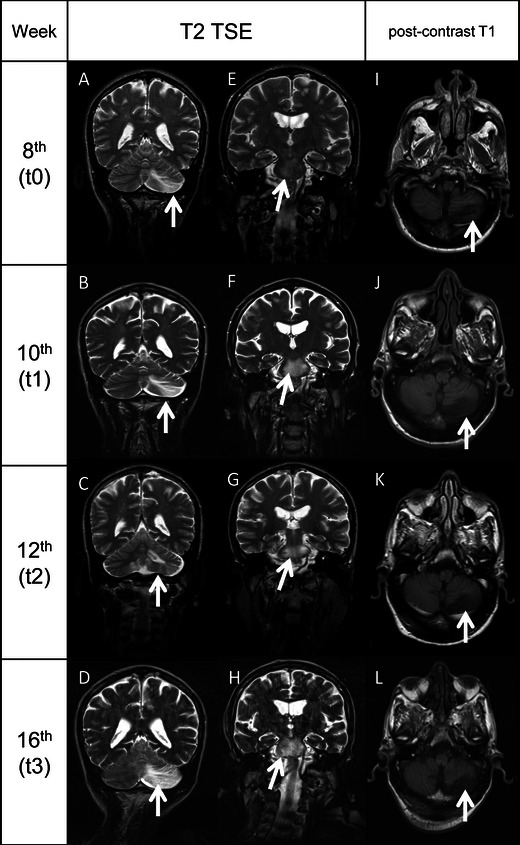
MRI evolution of the brain lesions. *A–D*, T2-TSE sequences showing the evolution of the left cerebellar lobe lesion (*arrows*) from t0 to t3. *E–H*, T2-TSE sequences showing the evolution of the lesion of the pons (*arrows*) from t0 to t3. No mass effect was seen in all the images collected. *I–L*, post-contrast T1-weighted sequences demonstrating the absence of contrast enhancement (*arrows*) in the left cerebellar lobe lesion (MRI). All the white matter lesions did not show enhancement in T1-weighted sequences after contrast administration. The lack of mass effect and contrast enhancement in all the MRI performed from t0 to t3 reduced the reliability of an IRIS-PML, which is usually characterised by a strong inflammatory infiltration of tissues, determining an alteration of blood–brain barrier which translates in penetration of contrast inside the lesions and oedema, which is the cause of mass effect
